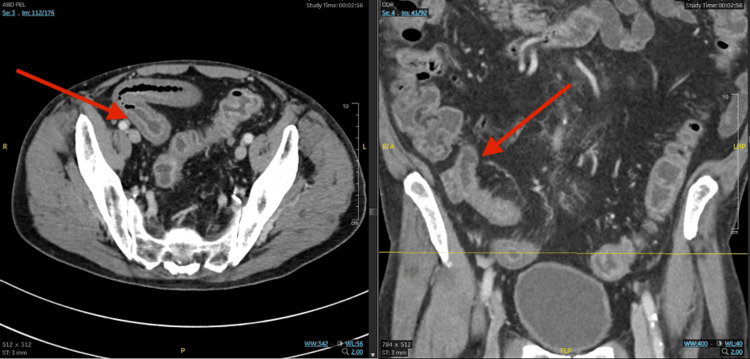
Coronal and sagittal abdominal CT scans in an amoebic colitis patient Diffuse inflammatory mural thickening of the terminal ileum and a diffuse fluid-filled colon are noted on the coronal and sagittal abdominal CT scans.
